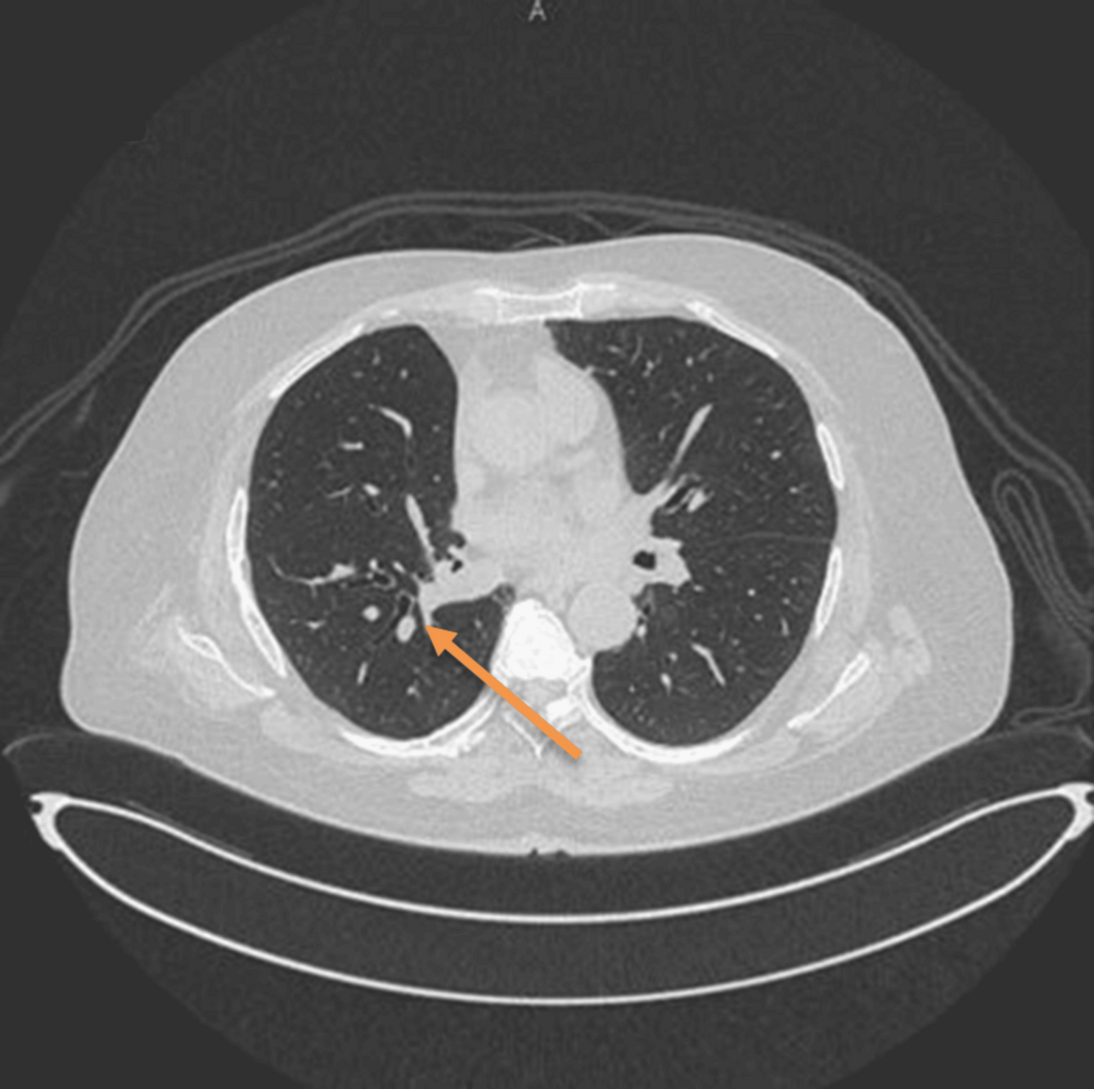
Thoracic CT imaging after lobectomy for pulmonary hydatid cyst with aspergilloma. Thoracic CT scan obtained one year after right upper and middle lobectomy demonstrating near-complete resolution of the prior cavitary lesion, with no evidence of residual disease or nodularity.
